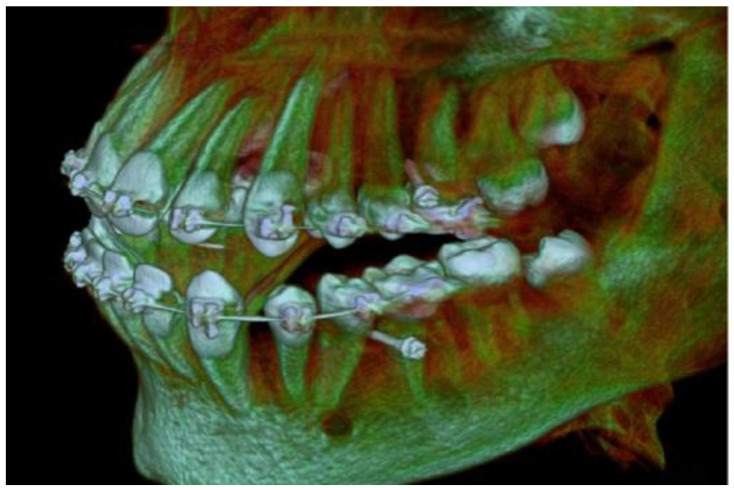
CBCT imaging during retraction with mini-implants in place.
